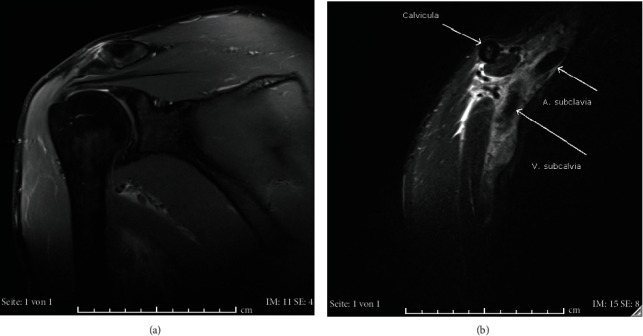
(a, b) MRI of the right shoulder. Siemens Symphony TIM. 1.5 Tesla. Syngo MR B 19. (a) Sequence: PD-TSE coronal, 3 mm TR 2950, TE 31, Acquisitions Matrix 0/384/296/0: shoulder MRI in the coronal plane without pathological findings. (b) Sequence: T2-STIR coronal, 3 mm, TR 6770, TE 35, IR 150, Acquisitions Matrix 0/384/296/0: patent subclavian vein without signs of thrombosis. Of note, MRI conducted in an external institution. Plane has been labelled as coronal, although it appears rather sagittal.
